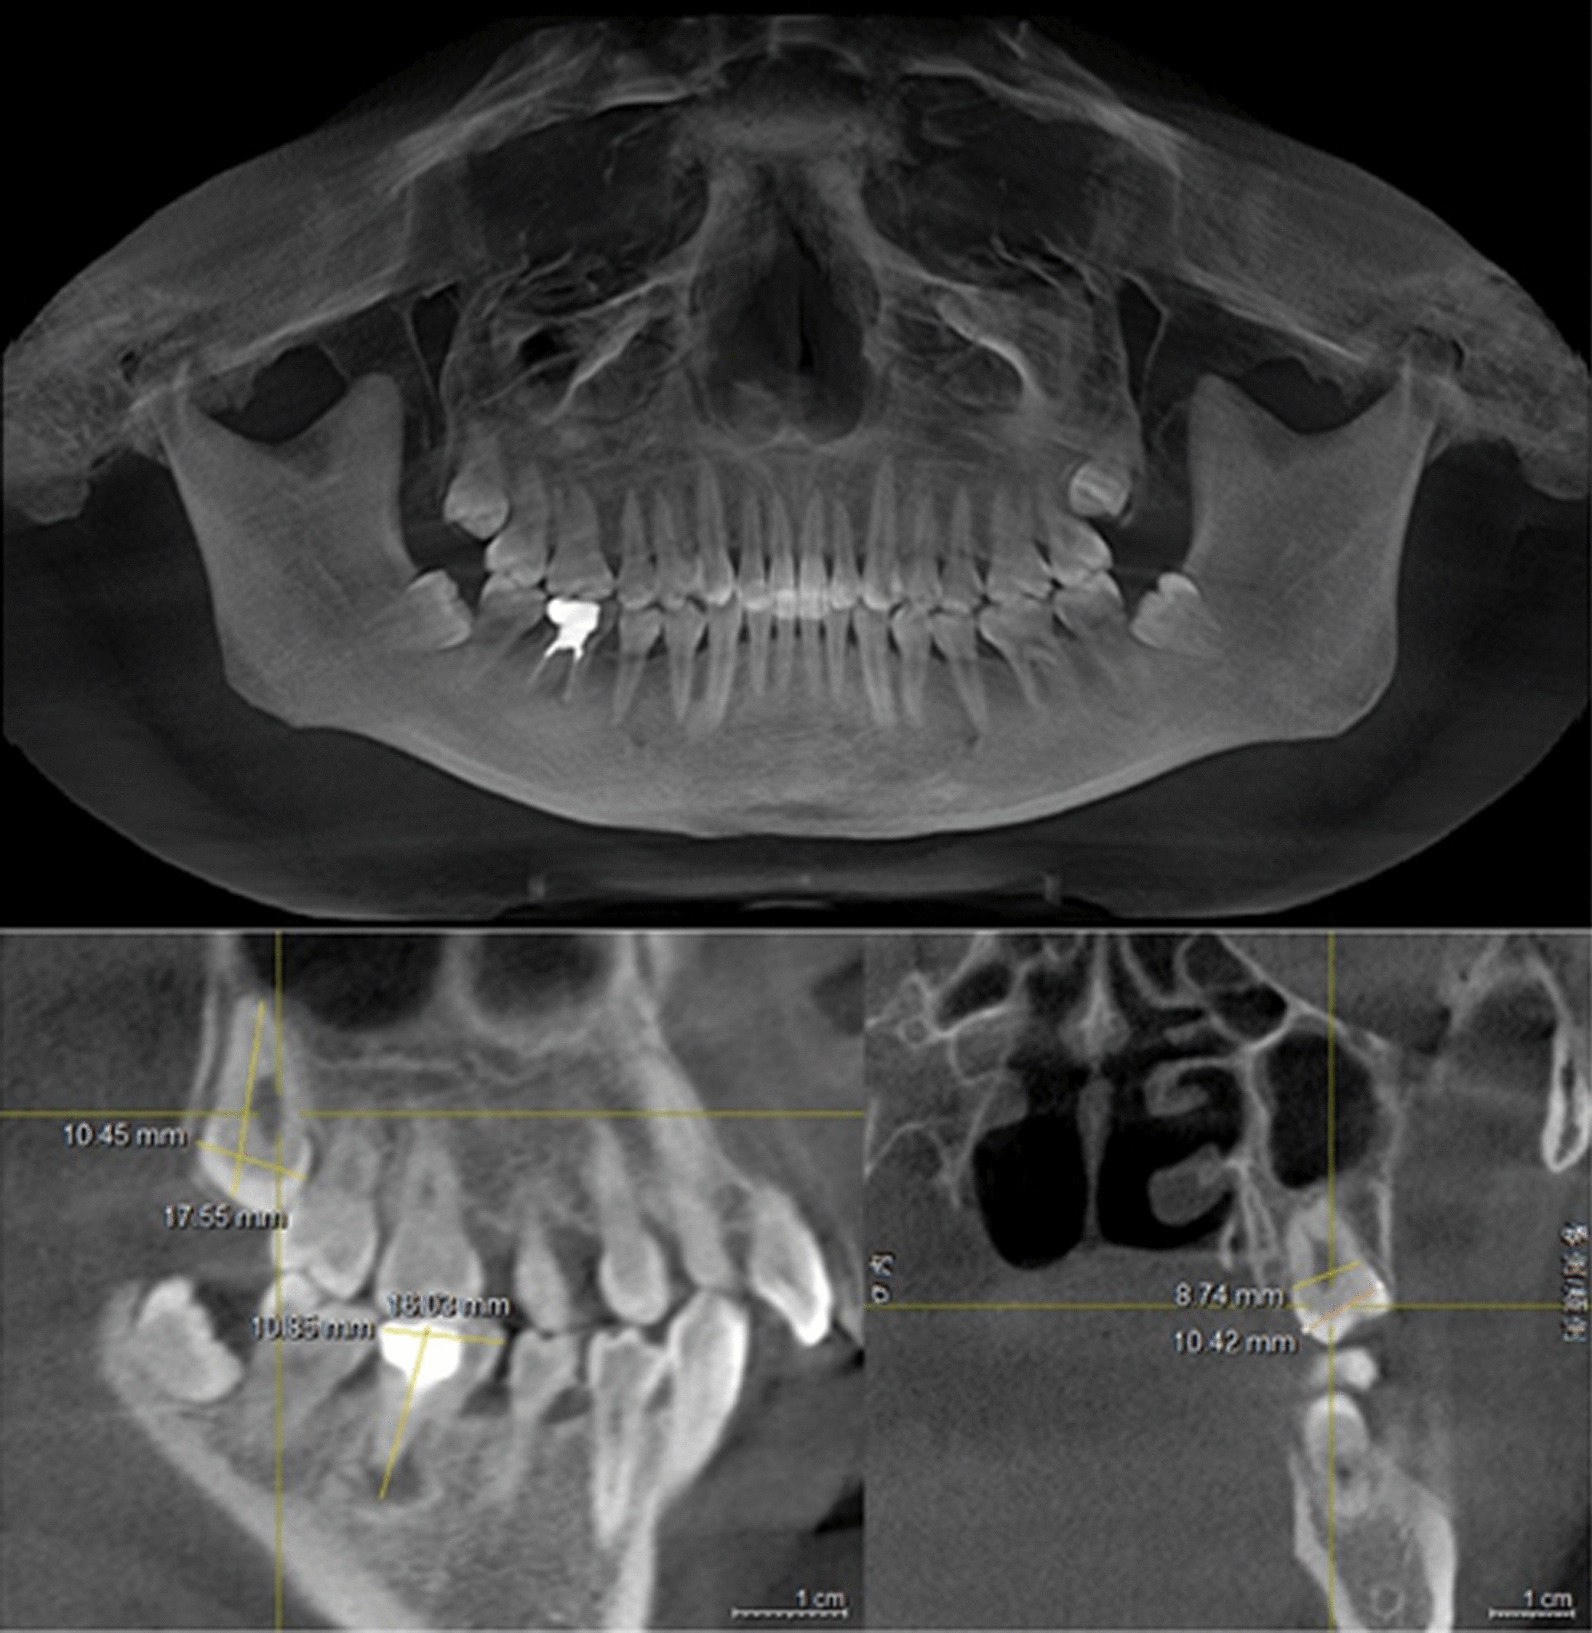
The measurements of the CBCT for preoperative evaluation. sagittal view: the measurements of right mandibular first molar (#46) and right maxillary third molar (#18); coronal view: the measurements of mesial/proximal and distal width of crown and root neck (#18)
